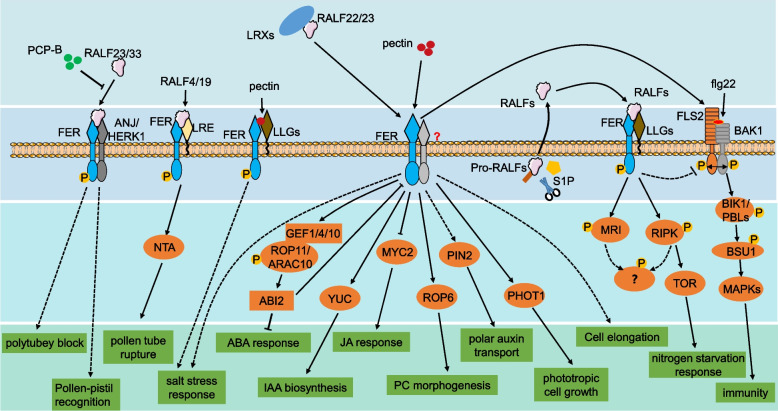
Model of FER signaling pathways. The FER signaling pathway could be modulated by a 4-layers mechanism. Firstly, the extracellular ligands (RALFs, pectin and PCP-Bs) and ligand combinations (LRXs) could trigger relatively specific FER signal activation. PCP-Bs compete with RALF23/33 to deactivate FER regulated polytubey block and pollen-pistil recognition. RALF4/19 and pectin activate FER mediated pollen tube rupture and salt stress response, respectively. RALF22/23 prefer to interact with cell wall-associated LRXs at acidic pH, and RALF22/23-LRXs complex could be disassociated under salt stress or alkaline conditions to release RALF22/23 and activate FER signaling pathway. Secondly, the diverse co-receptors(ANJ/HERK1, LRE and LLGs) provide diverse perception and responses mode for FER. FER forms a complex with ANJ/HERK1 to sense PCP-Bs and RALF23/33 signals. The FER-RALF4/19-LRE complex was formed upon recognizing RALF4/19 ligands. Moreover, FER form complexes with LLGs to sense pectin and RALFs. The third FER signaling modification layer was compromised of downstream components of FER. NTA, YUC, MYC2, ROP6, PIN2 and PHOT1 are downstream components for FER, which mediate FER regulated pollen tube rupture, IAA biosynthesis, JA response, PC morphogenesis, polar auxin transport and phototropic cell growth, respectively. The FER downstream GEF1/4/10-ROP11-ABI2 pathway was also revealed to regulate ABA response. MRI and RIPK are two RLCKs downstream of FER and RIPK directly interact with TOR to regulate FER related nitrogen starvation response. However, more RLCKs downstream of FER and more pathways downstream of the RLCKs remain to be discovered. Moreover, FER interacts with FLS2 to facilate the formation of FLS2-flg22-BAK1 complex and thus activate FLS2-BIK1-BSU1-MAPKs mediated immunity responses
